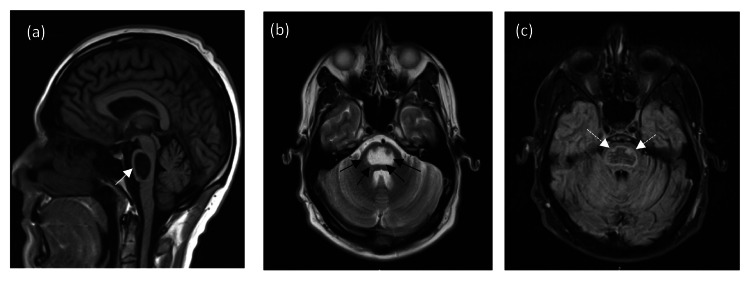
MRI brain: sagittal T1 (a) demonstrates central pons low signal intensity (white arrow). Corresponding axial T2 (b) shows high signal (between black arrows) and trident-shaped FLAIR (c) high signal intensity (dashed white arrows) surrounding the central low signal intensity in keeping with encephalomalacia changes. FLAIR: fluid-attenuated inversion recovery
